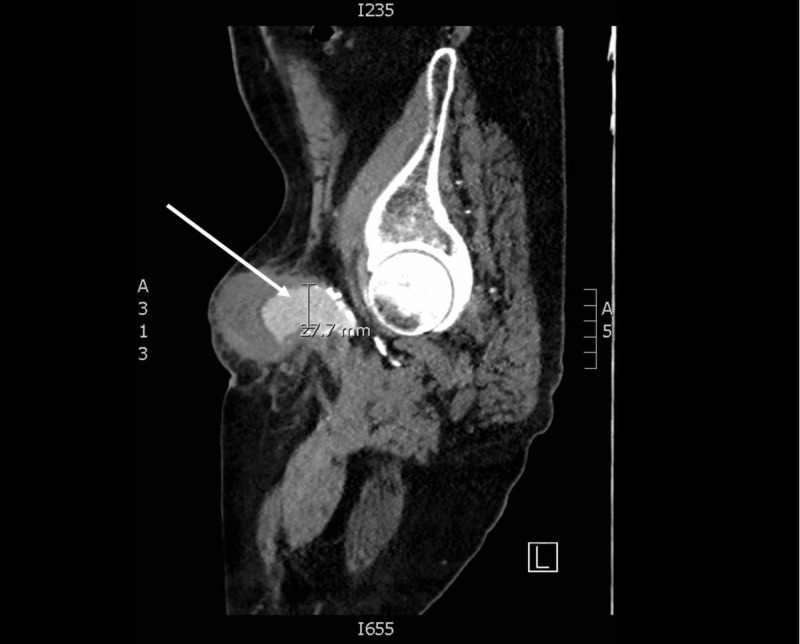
A sagittal slice of a computed tomography pelvis angiogram demonstrating 2.7 cm left common femoral artery at its widest diameter (solid white arrow)
